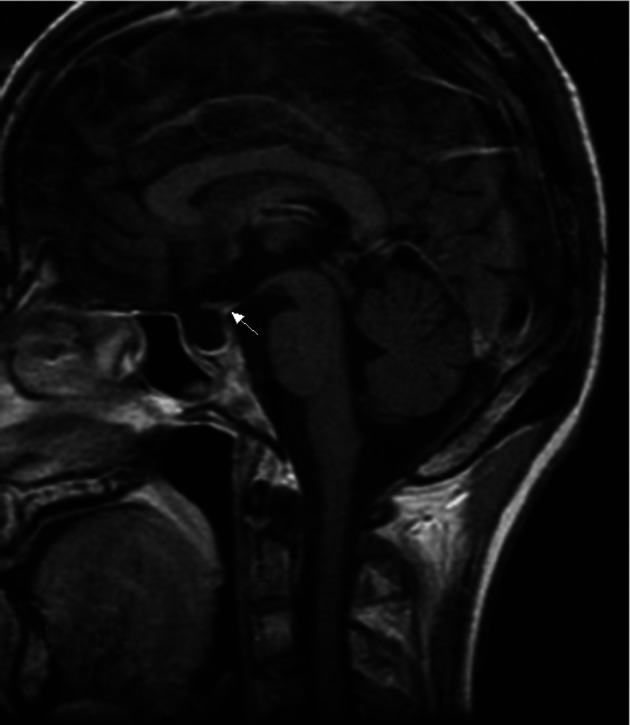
Enhanced magnetic resonance image (MRI) of the sagittal section of patient 1 showing the narrowing of the nodular lesions below the hypothalamus region following treatment.
